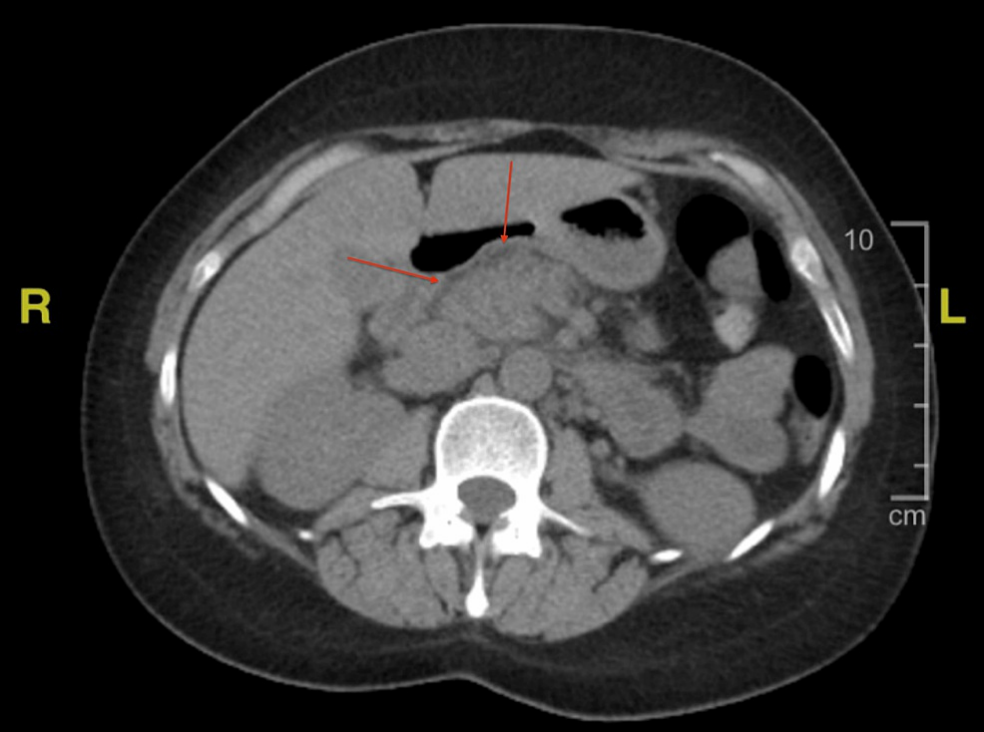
Upper abdominal CT scan without contrast shows peripancreatic edema consistent with acute pancreatitis.
